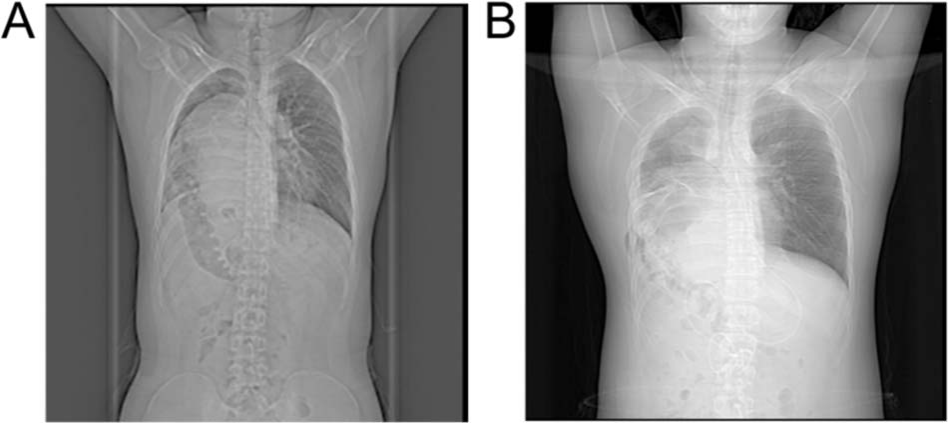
X-ray images: (A) Preoperative X-ray showing a suspected liver mass occupying the right thoracic cavity; (B) Postoperative X-ray showing re-expansion of the right lung and the liver returned to the abdominal cavity.
